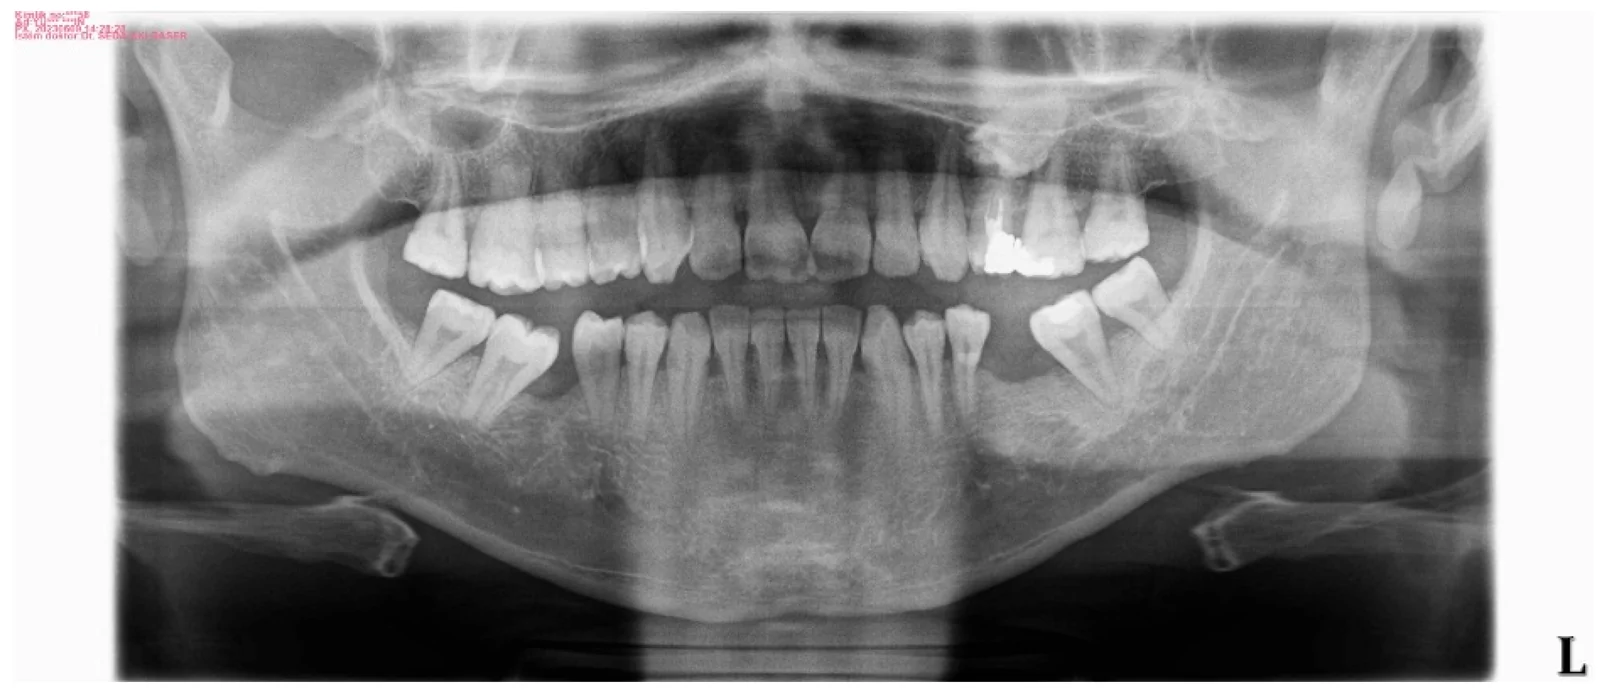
A panoramic radiograph of the mother of the patient is shown in Figure 3, demonstrating similar pyramidal molar morphology in the molar teeth.
